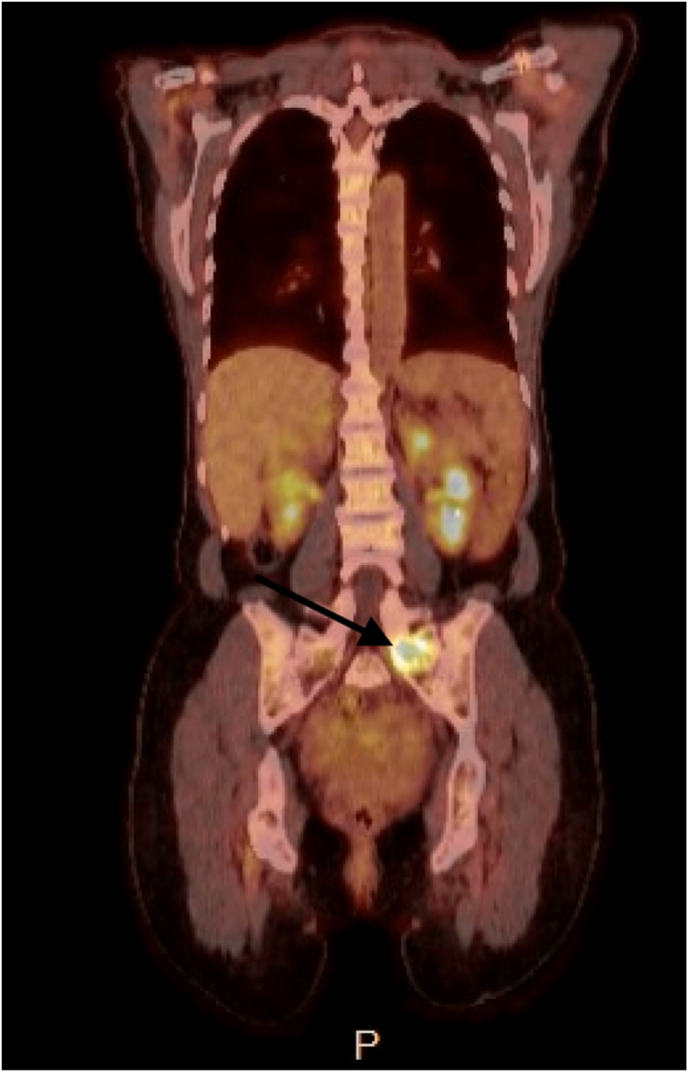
18-F-FDG PET/CT imaging with arrow pointing to FDG uptake in left pelvic sidewall lesion.
